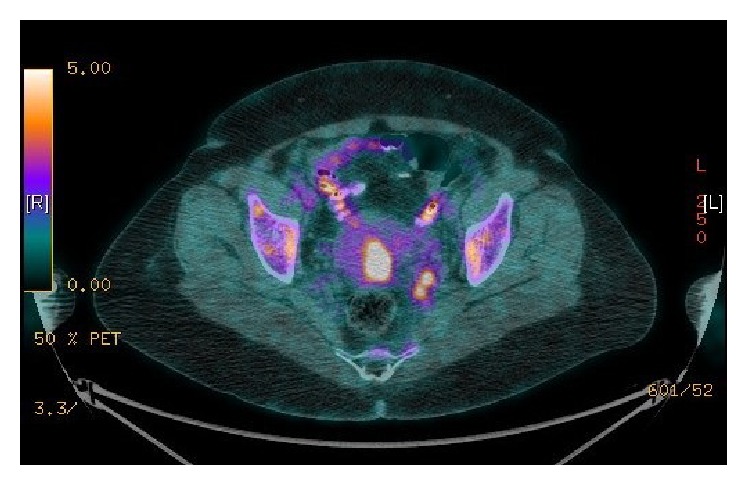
CT-PET imaging showing uptake in two left internal iliac lymph nodes and uptake in right external iliac small lymph node.
